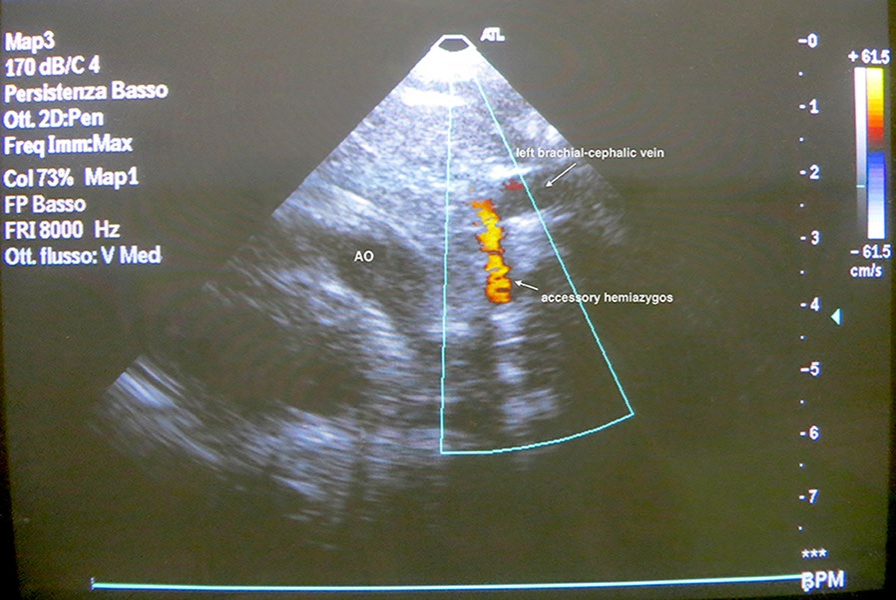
The postnatal echocardiography, through color—Doppler, shows the anterograde flow of blood draining in the left brachiocephalic trunk, as reconstructed in anatomical model in Fig. 1
